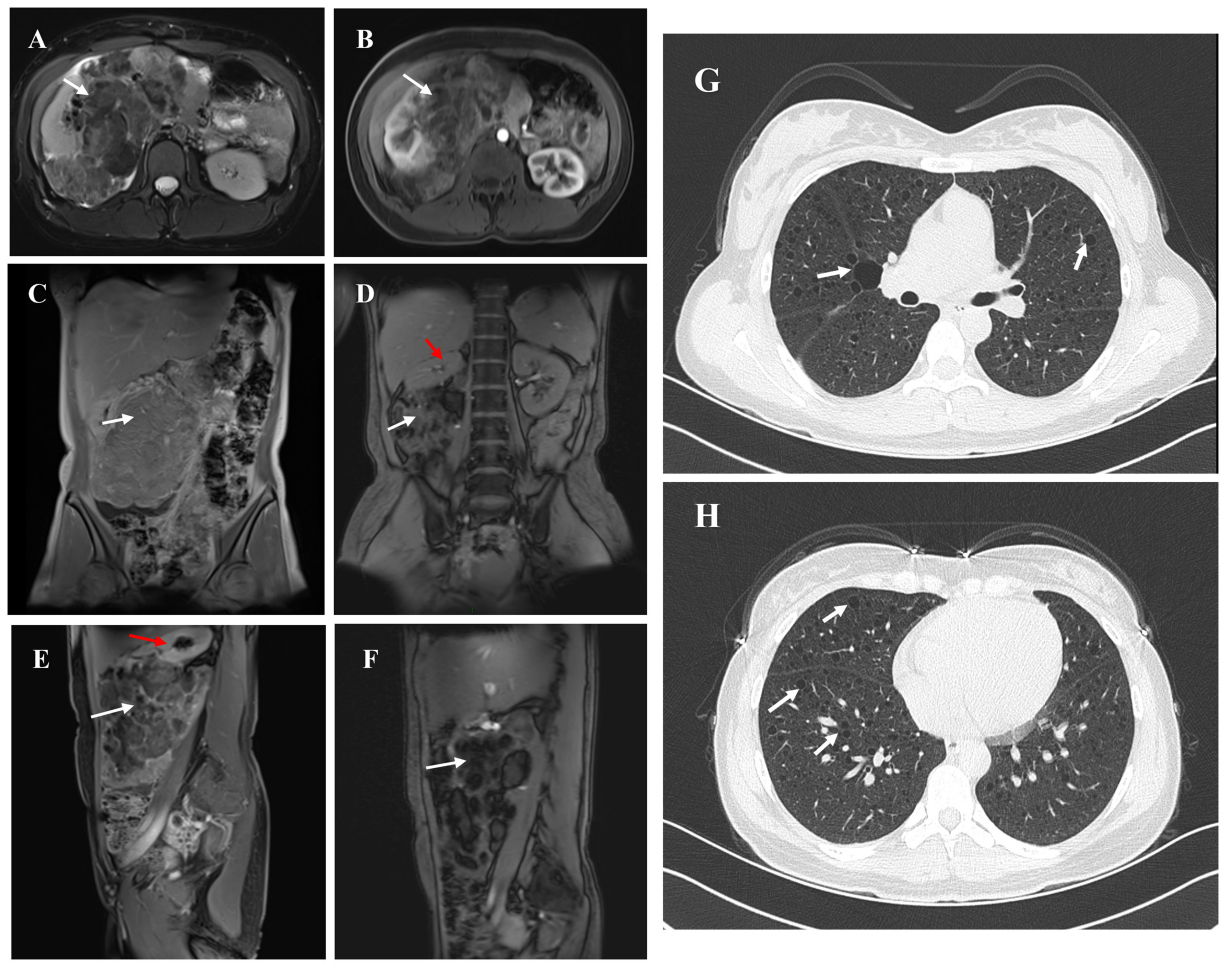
The figure summarizes the findings from imaging examinations of the primary tumor lesion. (A, B) Non-contrast AND Arterial phase contrast-enhanced MRI. White arrow indicates right renal angiomyolipoma and red indicates the remaining normal tissue of the right kidney. (C–F) Coronal/Sagittal MRI showing a giant right renal angiomyolipoma. (G, H) Chest CT. White arrow indicates the Thin-walled cystic lesions in both lungs.
